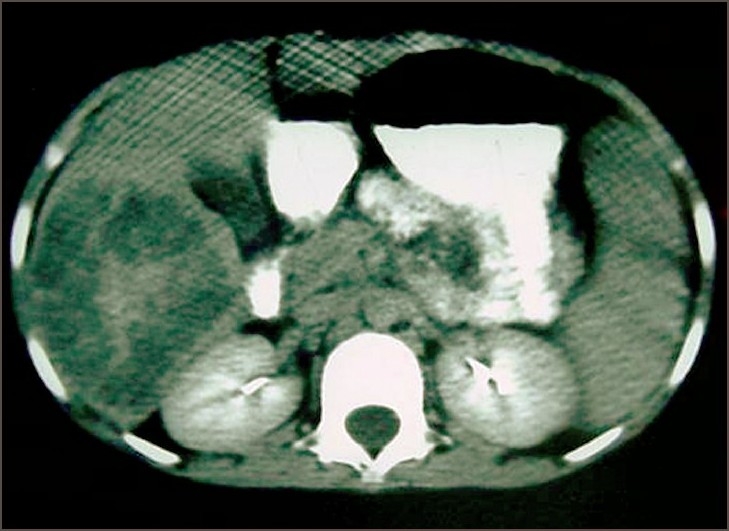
CT scan shows large irregular solid heterogenous enhancing mass in the right lobe of liver
